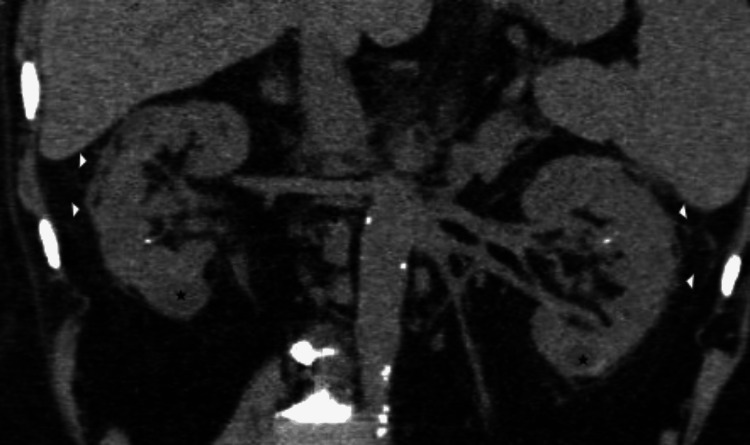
Acute pyelonephritis on non-contrast CT. A 43-year-old woman presenting with an acute history of fever and abdominal pain was diagnosed with acute pyelonephritis. Coronal reformatted non-contrast CT of the abdomen image shows bilateral enlarged kidneys with perinephric fat stranding (arrowheads) around both kidneys. A few renal calculi and well-defined hypodense cortical cysts (black asterisks) are also seen in both kidneys. These images are from the hospital and have not been published elsewhere. CT: computed tomography
